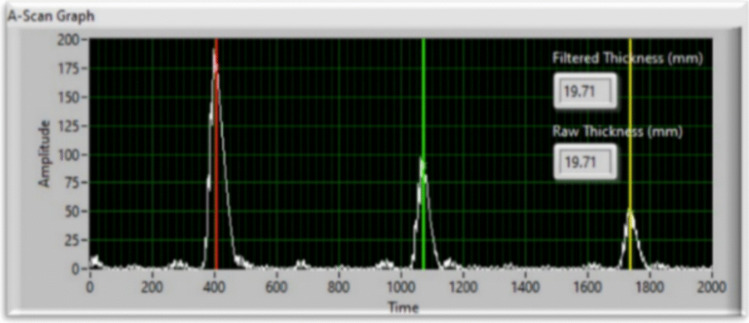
Ultrasonic A-scan signals
